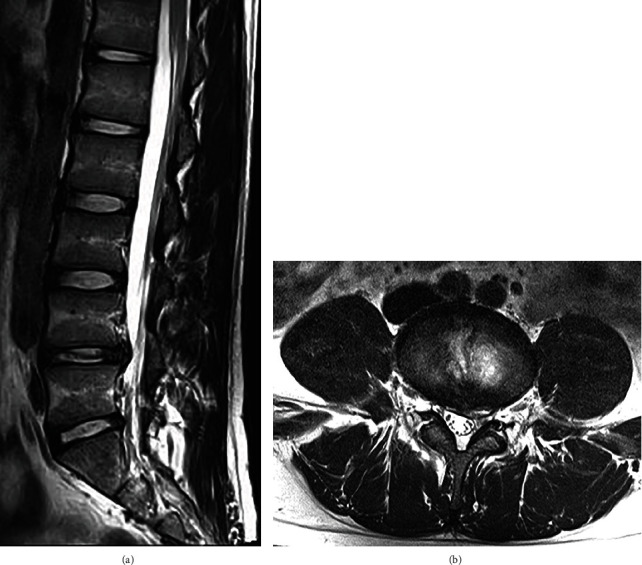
Magnetic resonance imaging before transforaminal full endoscopic lumbar discectomy 8 years ago. A (a) sagittal section and (b) horizontal section of the L4/5 showed subligamentous lumbar disc herniation.
